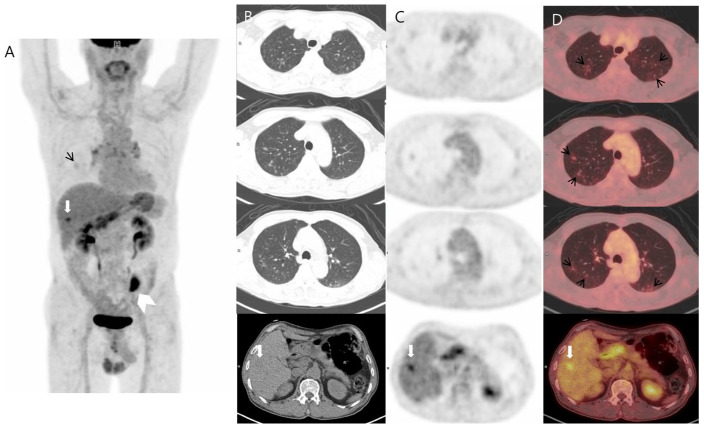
Three-dimensional maximum intensity projection (A) and transaxial images ((B) CT; (C) PET; (D) fusion) of ^18^F-FDG PET–CT demonstrated, in addition to a hypermetabolic mass in the sigmoid colon (white arrowhead), abnormal multiple cavitary pulmonary nodules with mildly increased FDG uptake (black short arrows) and a focal hypermetabolic nodule in the right hepatic lobe (white arrow). The maximum standard uptake values were 1.81 for the pulmonary nodule and 4.8 for the liver lesion.
